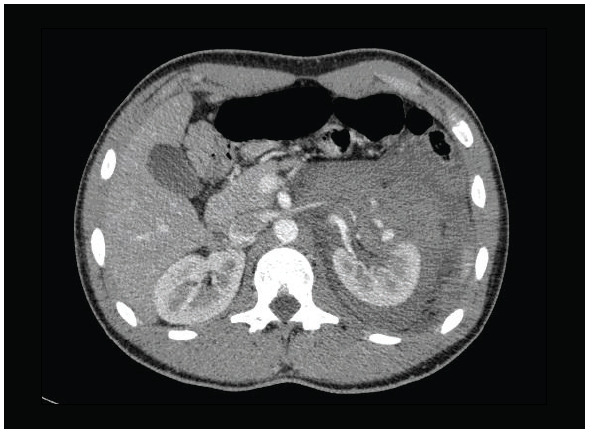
Computed tomography (CT): grade III left renal injury with large perirenal hematoma in Case 1.
